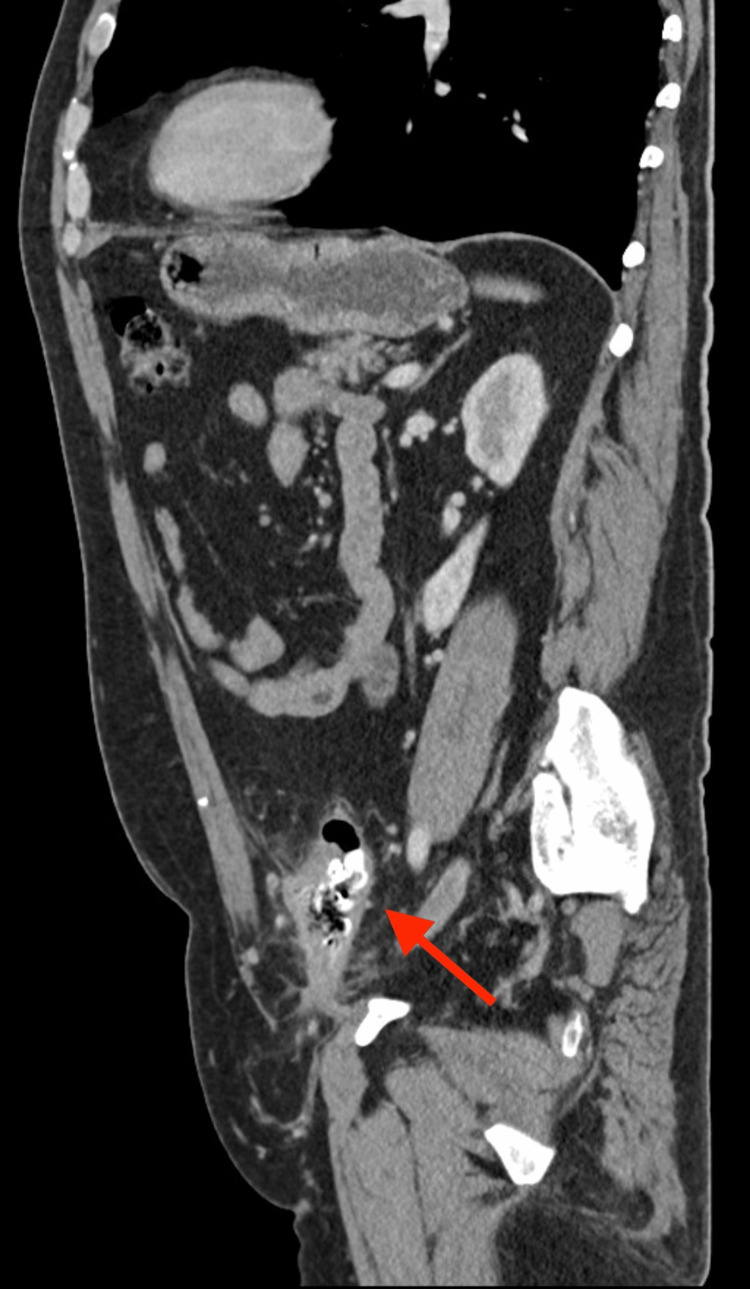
Abdominal CT scan (sagittal plane) showing foreign body (arrow) partially in the colon lumen and intraperitoneal collection with air
